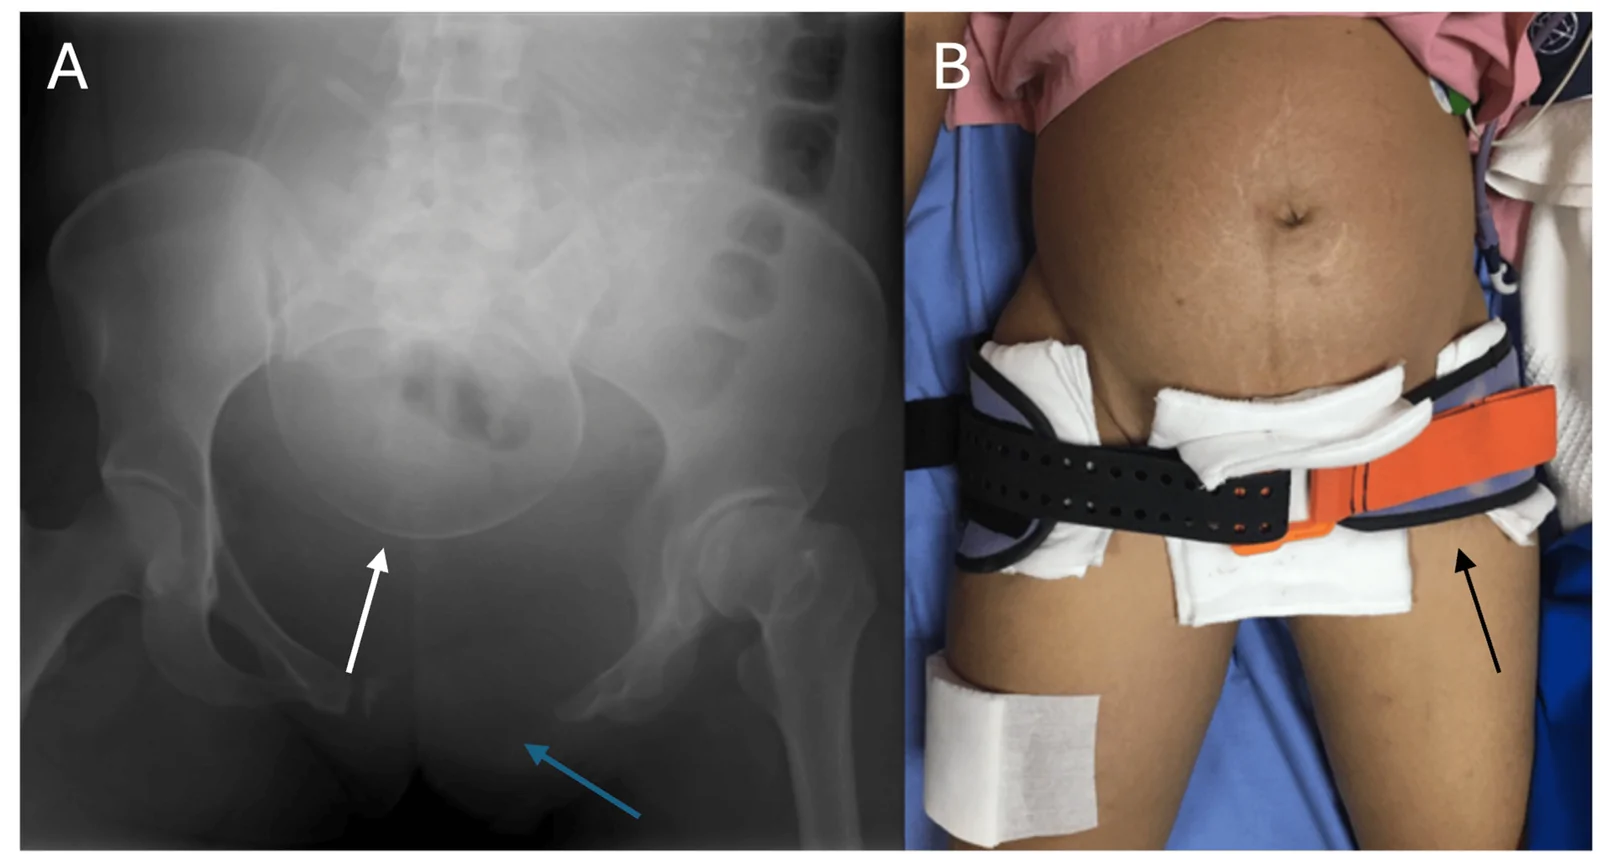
Pelvic radiograph showing open-book pelvic injury and pelvic binder application (A) Anteroposterior pelvic radiograph demonstrating an open-book pelvic injury (Young-Burgess anterior-posterior compression type II (APC-II)) (blue arrow), with the fetus in vertex position (white arrow). (B) Pelvic binder (black arrow) applied for provisional stabilization.
